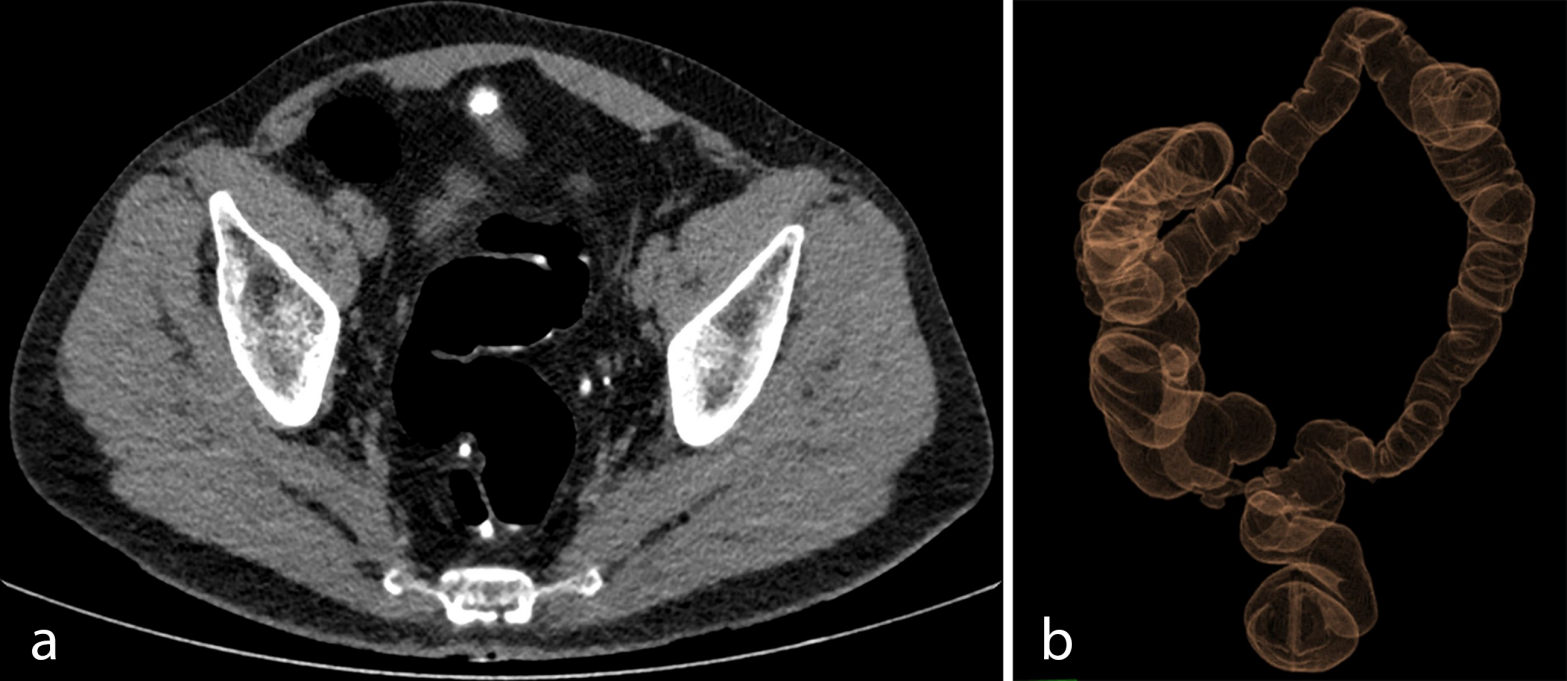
CTC images obtained after surgical intervention: (a) axial section on anastomosis site and (b) CT 3D colon map.
